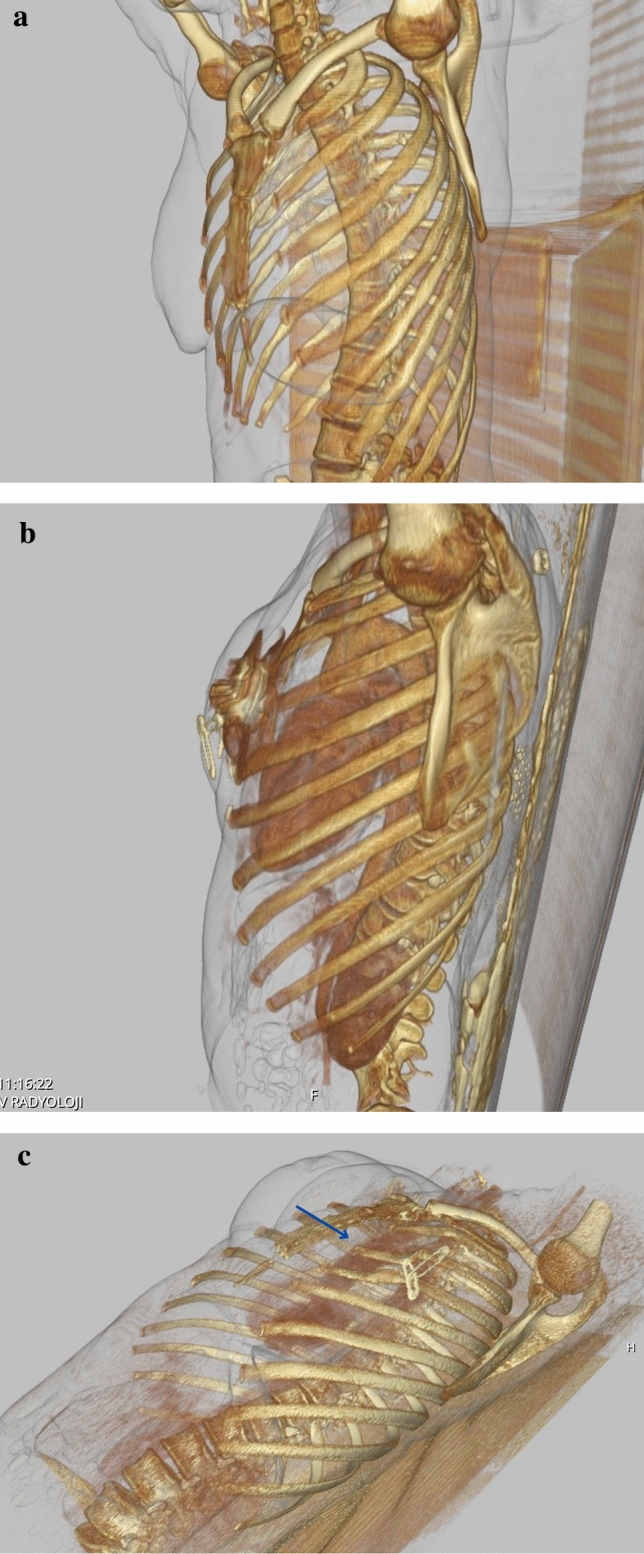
In the images above, you can see the 3D thorax CT of the same patient in the preoperative (a), early postoperative expander 1st-stage (b), and early postoperative expander 2nd-stage (c) periods. The remodeling in the 3rd rib corresponding to the center of the expander is clearly visible (blue arrow)
